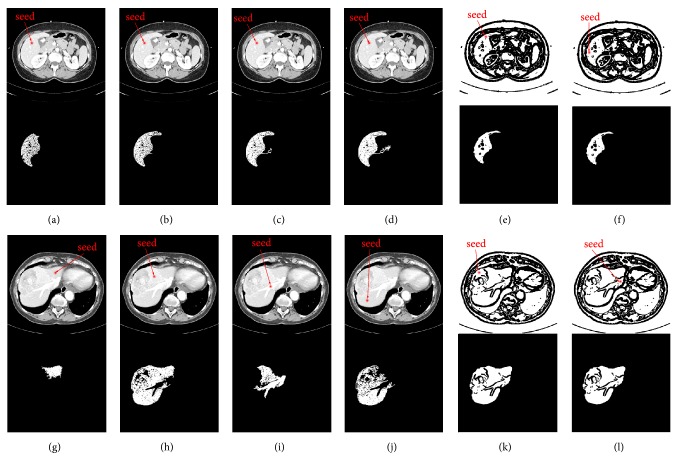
The comparison between region-growing for original CT image and region-growing for binary image. (a)-(b) indicate the original image M, (e)-(f) indicate the binary image of image M, (g)-(j) indicate the original CT image N, and (k)-(l) denote the binary image of image N. And images below (a)-(l) denote the corresponding results of region-growing. The initial seed points are denoted by red points, and *W* values of (a)-(d) were set to 0.04, 0.05, 0.06, and 0.07, respectively. *W* values of (g)-(j) were all set to 0.08, *W* values of (e) and (k) were both set to 0.3, and *W* values of (f) and (l) were both set to 0.8.
